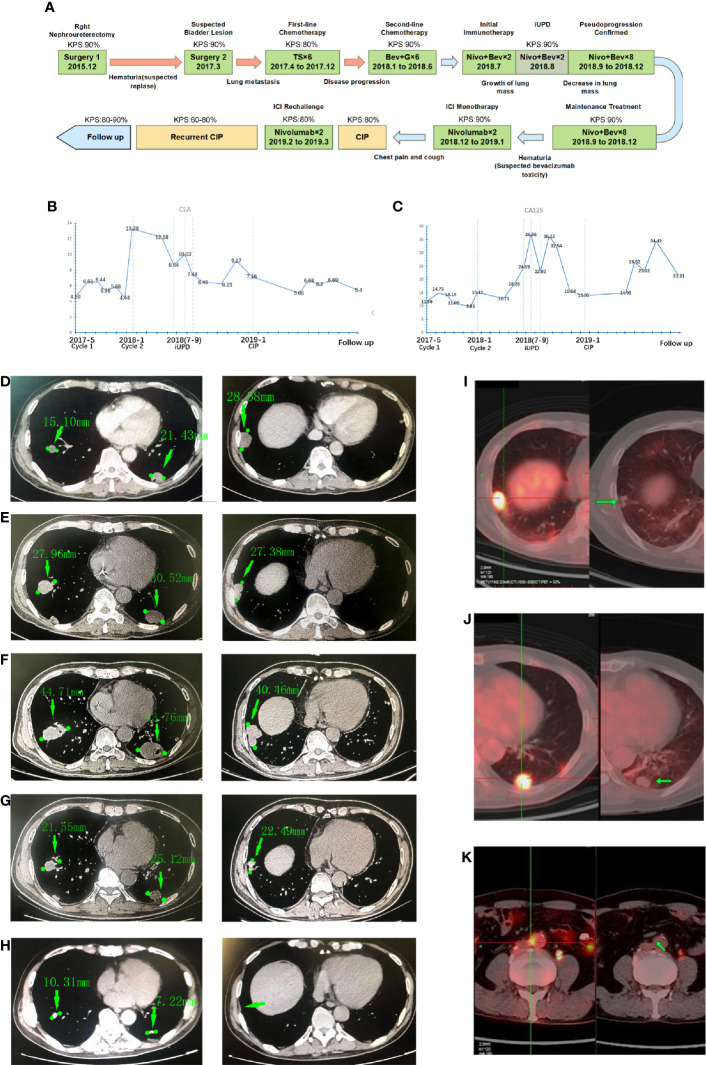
The time line, biomarker and medical imaging information of the patient. (A) Time axis of antitumor treatment and intervention in terms of side effects. (T = paclitaxel; S = S-1; G = gemcitabine; Bev = bevacizumab; Nivo = nivolumab), Karnofsky Performance Status (KPS) refer to the Karnofsky performance status during that period. (B) Carcinoembryonic antigen (CEA) levels during treatment; (C) CA125 levels during treatment. Chest CT images showing the tumors on lungs: (D) The initial images at the time of diagnosis showed multiple pulmonary metastases and bronchial obstruction; (E) The images of the same lesion before the immunotherapy; (F) The pulmonary metastases were enlarged transiently on the first follow-up examination; (G) The lesion was decreased after the subsequent two cycles of treatment; (H) Further tumor shrinking on the latest follow-up images, the lesion in right inferior lobar almost disappeared. Positron emission tomography (PET)/computed tomography (CT) of the patient: The left panel of each picture shows the initial image of the lung metastases, and the right panel shows the images of the same lesions 10 months after the termination of immune checkpoint inhibitor (ICI) treatment: (I) Metastatic tumor in the right lung; (J) Metastatic tumor in the left lung; (K) Paraaortic node metastases and lesions disappeared after treatment.
